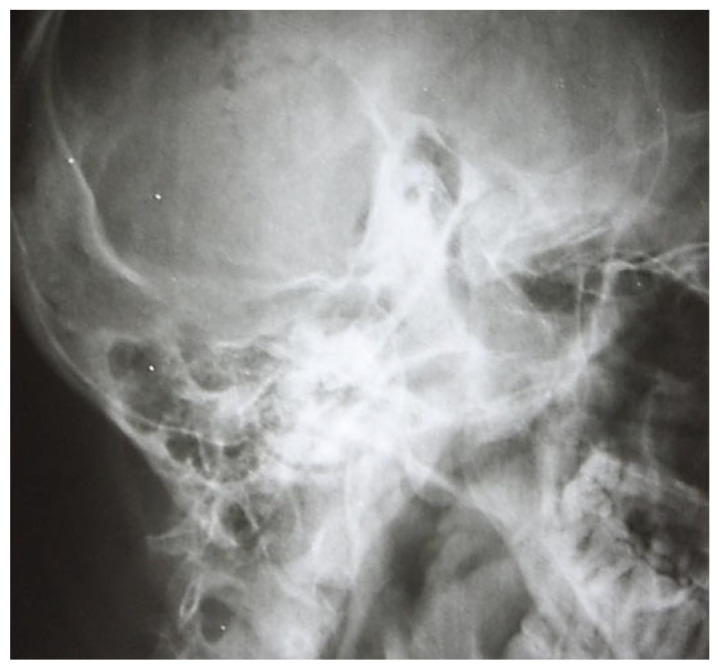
Postoperative control radiograph showing an area of osteolysis with clear, well-demarcated borders.
